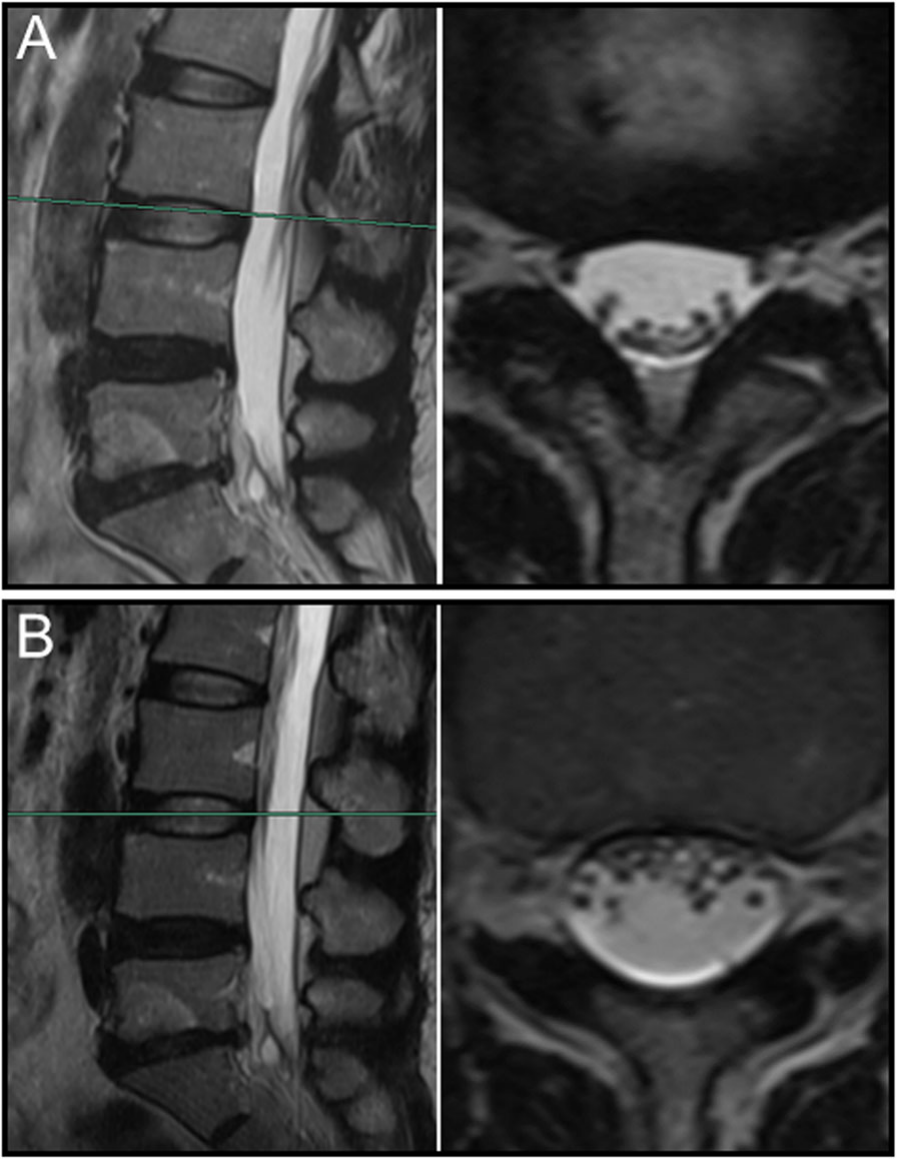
In a woman with negative SedSign (a), the nerve roots sedimented to the ventral side of dural sac on prone MR images (b)
